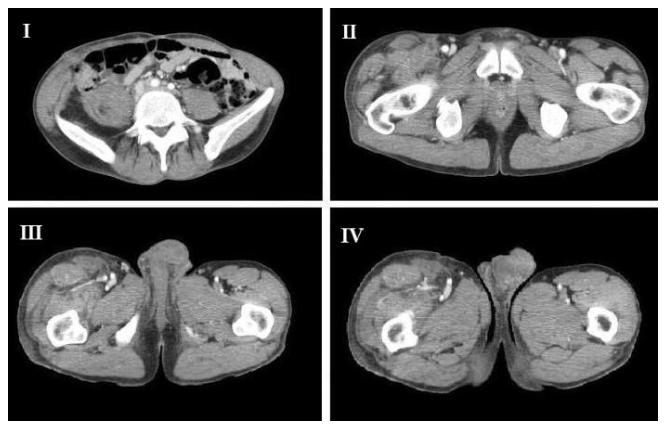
Postoperative computed tomography showing the outcome of the treatment. The psoas muscle has been spared despite the resection of the cysts (I, II); the right thigh is free of cysts with the conservation of the muscular structures (III, IV).
